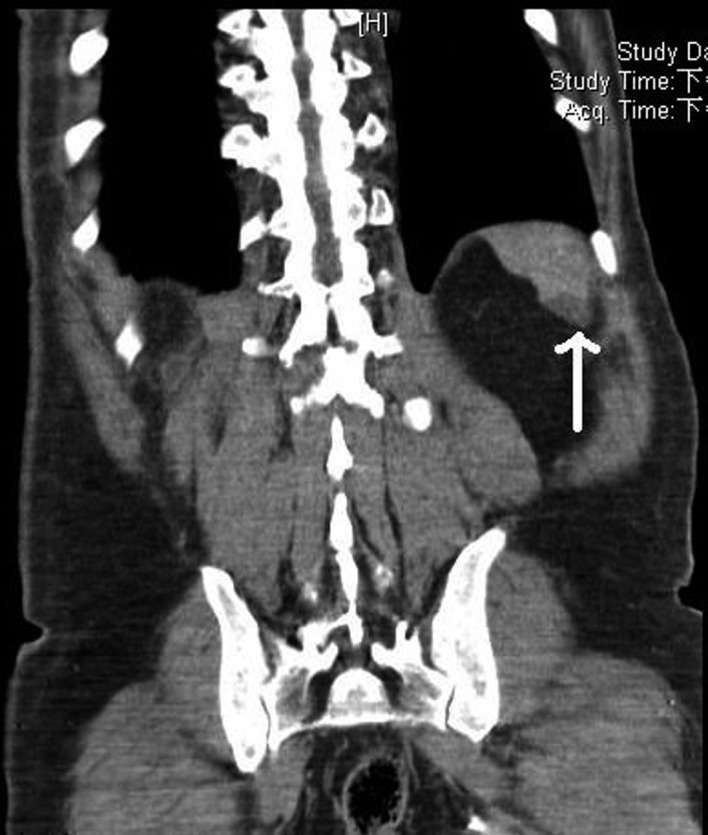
Abdominal CT indicating a nodular lesion at the spleen (point by white arrow) that was 1.6 cm in diameter and showed mild enhancement in a post-contrast study, indicative of a spleen abscess or infarction.
